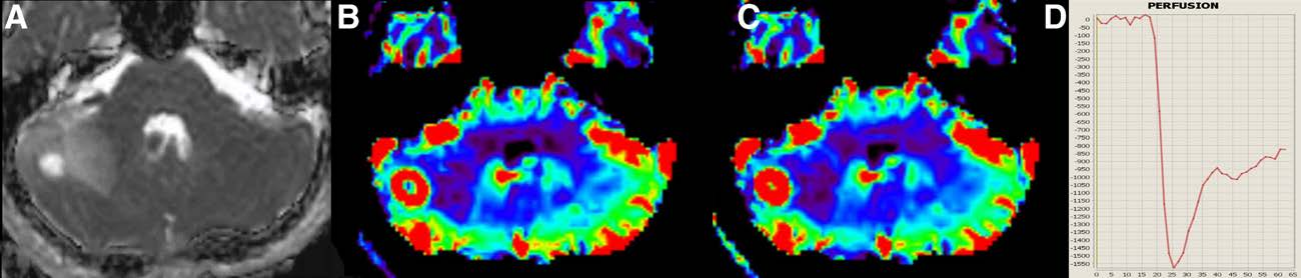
Hemangioblastoma in a 25-year-old man. MRI shows an oval mass in the right cerebellar hemisphere. Normalized mean apparent diffusion coefficient is 1.17 (a, arrow). Normalized relative cerebral blood flow and volume are 5.53 and 8.97, respectively (b and c, arrows). The percentage signal recovery is 43.0 (d). MRI = magnetic resonance imaging.
